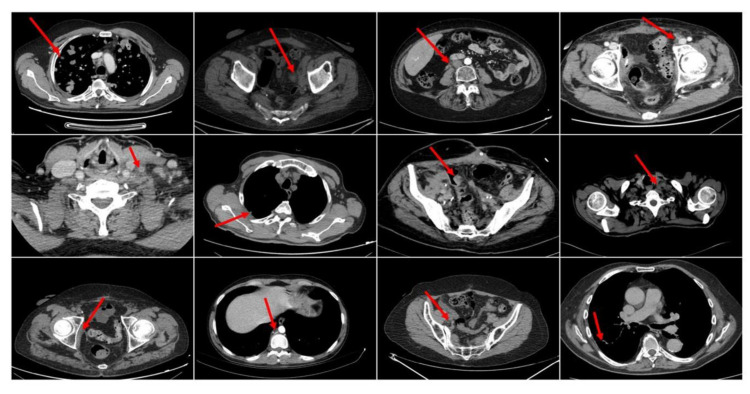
Some instances of the selected RECIST (Response Evaluation Criteria in Solid Tumors) compliant cancer lesions in Computerized Tomography (CT) imaging. The red arrow identifies an instance of the selected RECIST 1.1. compliant lesion.
